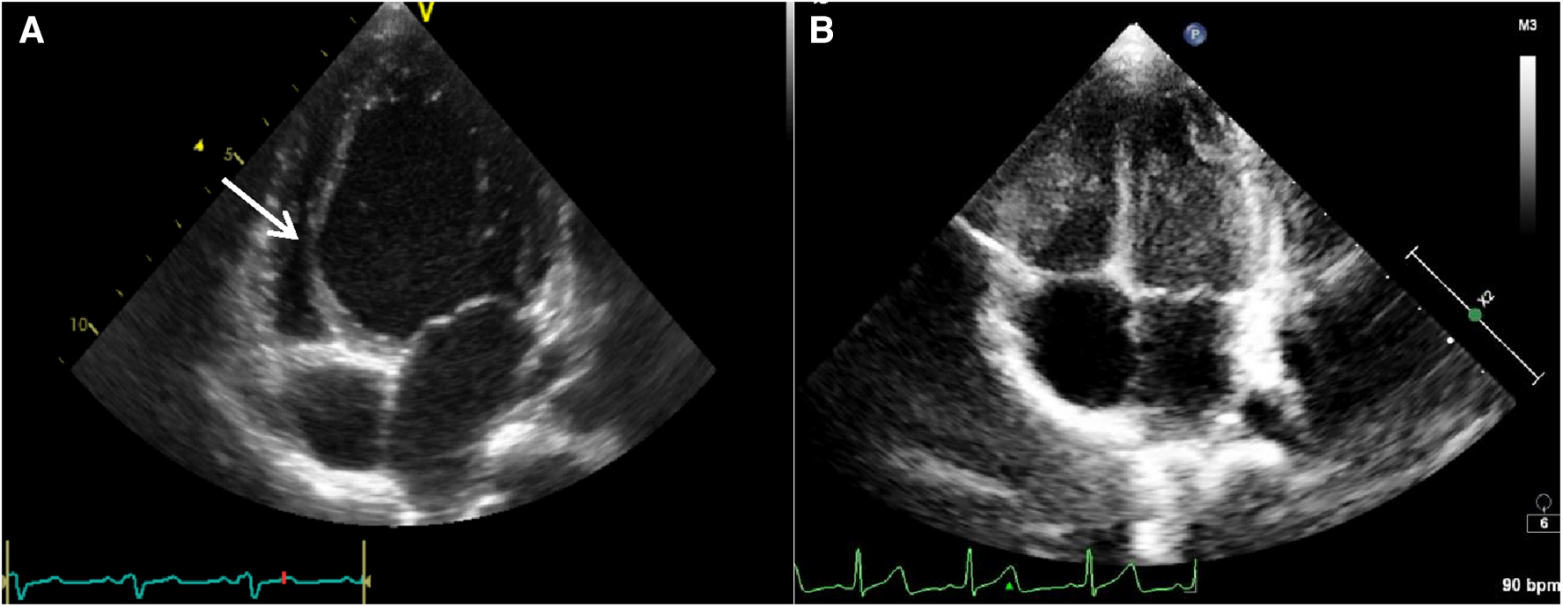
The parasternal long axis four-chamber view of case 1 showed the left ventricle was spherically dilated and the basal/middle segment of the interventricular septum (IVS) contracted paradoxically like an aneurysm (see the arrow) protruding into the compressed right ventricle before pharmacotherapy for cardiac resynchronization (A). The paradoxical motion of the interventricular septum disappeared and the diameter of LV returned to normal after successful pharmacotherapy for cardiac resynchronization (B).
